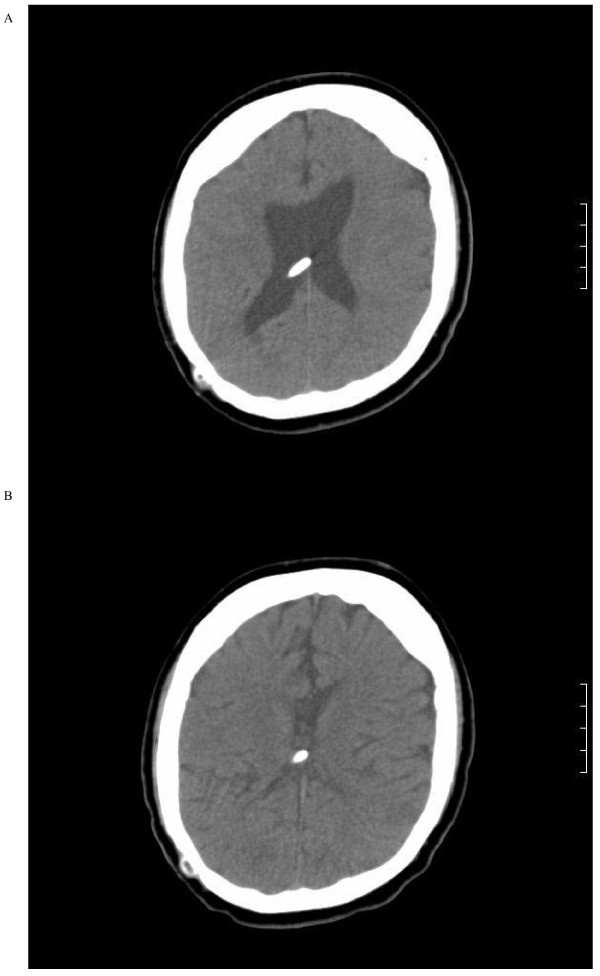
CT scan of the patient's brain. (A) Preoperative ventricular dilatation. (B) Postoperative decompression of the ventricle
